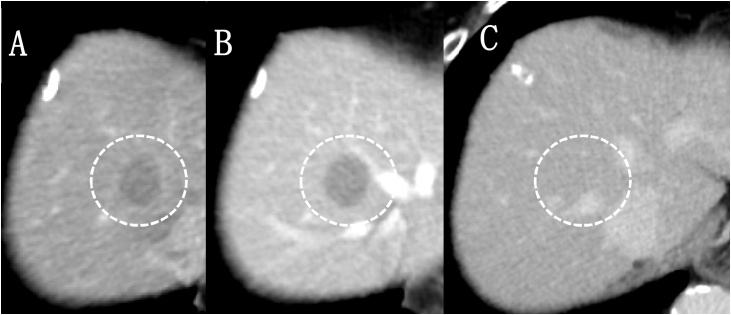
Dynamic abdominal computed tomography findings A: The arterial phase showing a ring-like enhanced mass at segment 7 measuring 35 mm in diameter, shaped like an irregular arrow. B: Portal phase. C: The enhancement is prolonged to the late phase.
